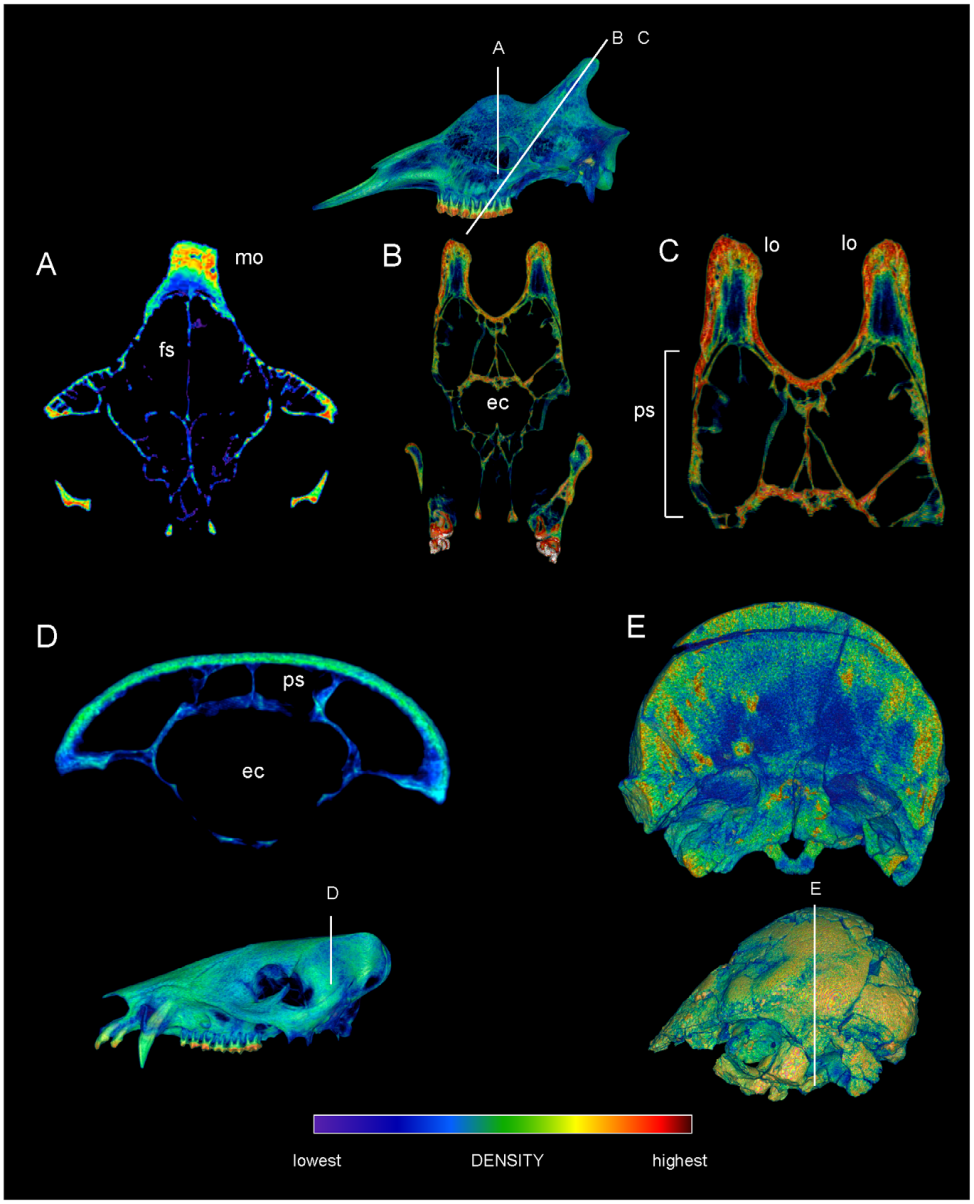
Cranial densities in *Giraffa* (TMM M 6815, UCMZ 1976.33), the peccary *Tayassu* (UCMZ 1975.279), and pachycephalosaur *Prenocephale* (GI SPS, field number PJC2004.8). CT sections through crania of comparative taxa, with slices mapped onto lateral renders of crania. A. *Giraffa camelopardalis* male (TMM M6815), transverse section through the region of a median ossicone. B. Oblique transverse section of *Giraffa camelopardalis* (UCMZ 1976.33) through the posterior ossicones. C. Enlargement of B focusing on the ossicones. The layering of densities in the giraffe ossicones resembles that in the dome of *Stegoceras validum* (Figure 6). D. Transverse section through the cranium of *Tayassu tajacu*, showing a non-cancellous skull roof over cranial sinuses. D. Section through the cranium of the pachycephalosaur *Prenocephale prenes* (GI SPS). Despite mineral inclusions (localized red and yellow areas) and CT artifacts, the scan shows dense superficial and cancellous deep regions within the dome. Abbreviations: ec = endocranial cavity, fs = frontal sinus, lo = lateral ossicone; mo = median ossicone; ps = parietal sinus.
